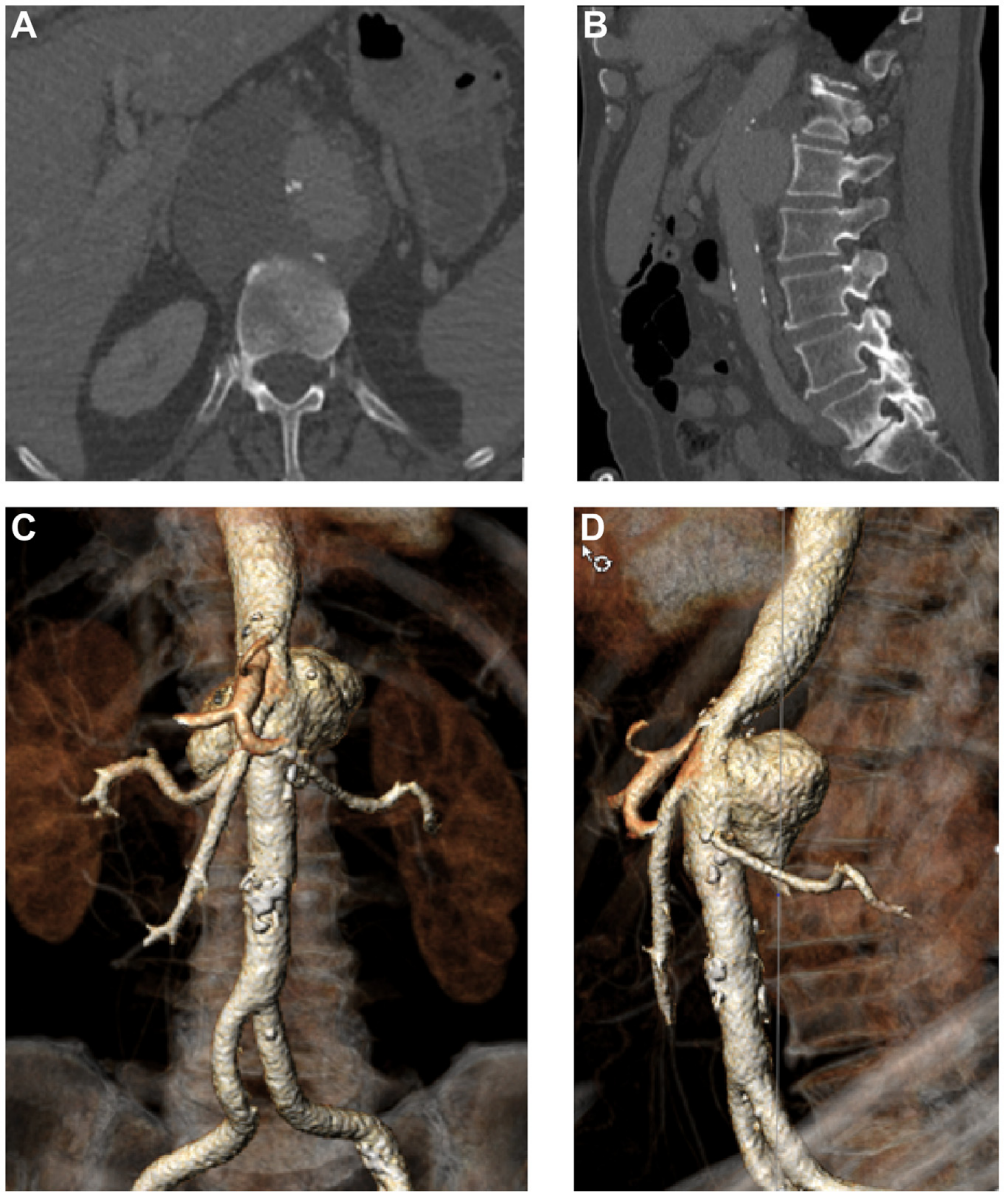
Preoperative contrast-enhanced computed tomography (CT) showing in an axial view (A) a rupture of a suprarenal aortic aneurysm and a retroperitoneal hematoma, and (B) in the sagittal view. (C and D) Corresponding three-dimensional reconstructions using *3mensio* software.
